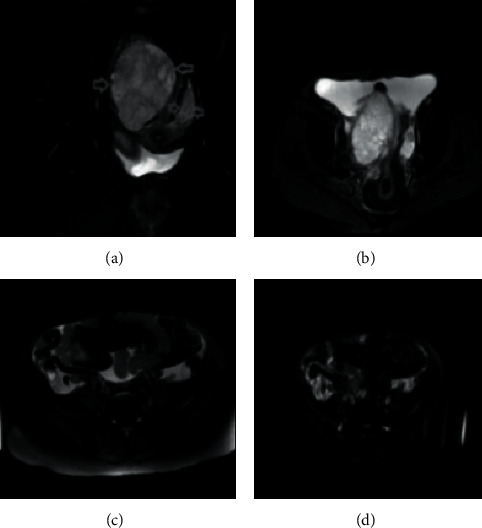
ADNEX combined with MRI in the same case for nuclear magnetic examination.
